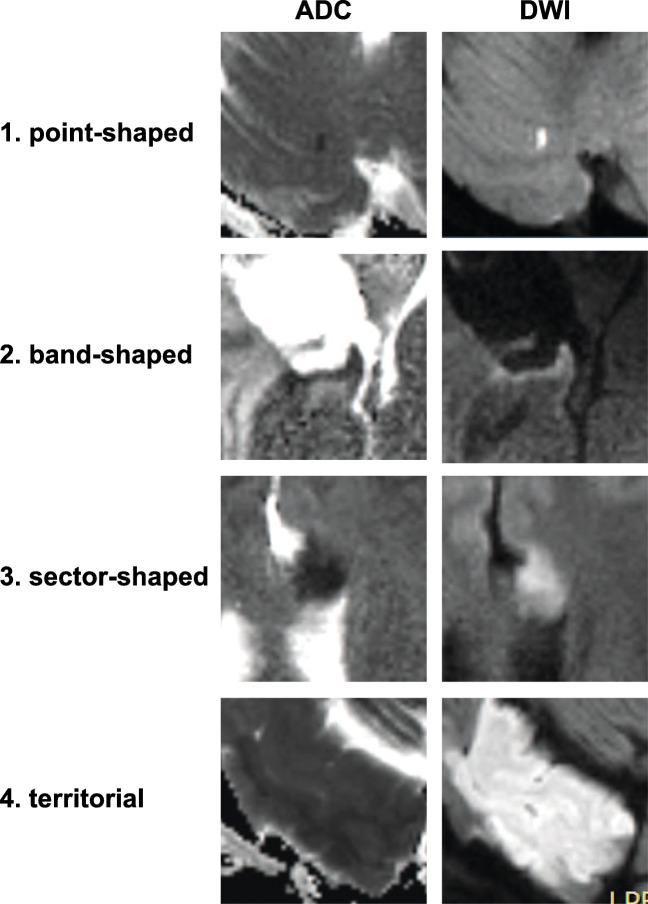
Examples of different infarct categories with their corresponding ADC and DWI images. 1. Point-shaped infarct; 2. thin band-shaped infarct at resection cavity; 3. sector-shaped infarct with involvement of deeper parts of the parenchyma; 4. territorial infarct. ADC apparent diffusion coefficient, DWI diffusion-weighted imaging
